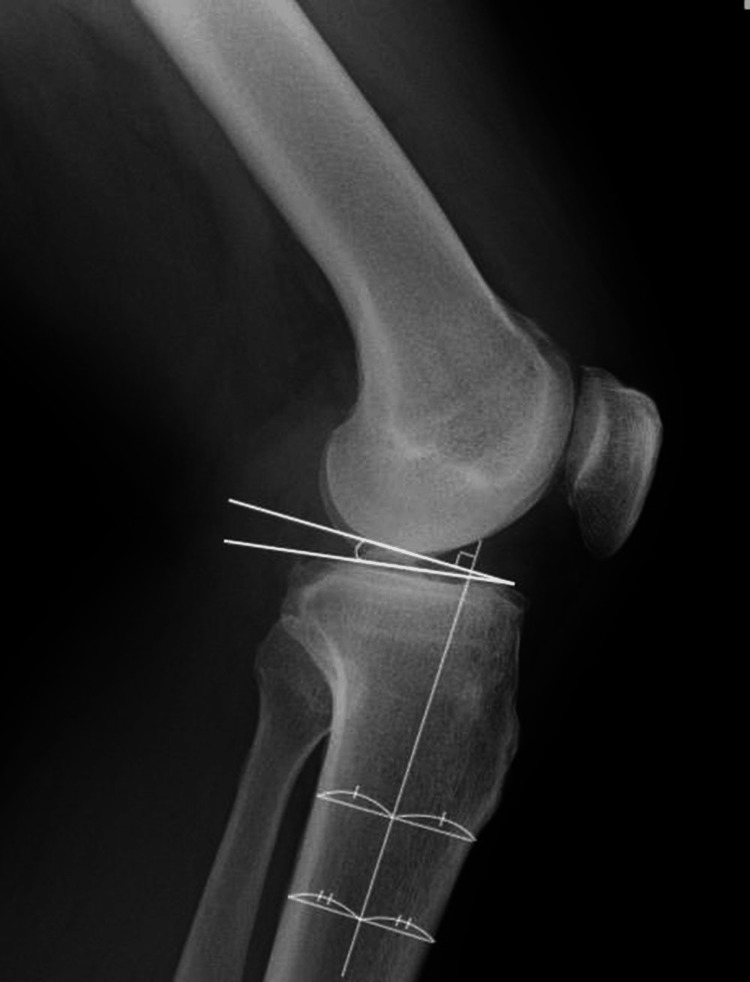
Lateral radiograph of the knee Seen is the PTS, the angle between the averaged slope of the medial and lateral tibial plateaus and a line representing the tibial diaphyseal axis. PTS: Posterior tibial slope Image credit: Author Tetsuro Shimada
